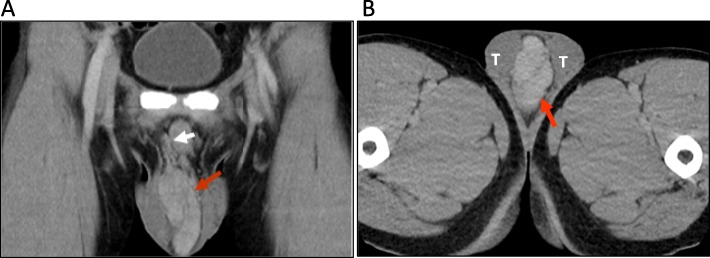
CT image demonstrating. (a) A 4.7 × 8.5 × 3.5 cm tumor (red arrow) with vascular supply (white arrow) arising from the right spermatic cord. (b) An extra-testicular mass (red arrow) within the scrotum between the two testes (T)
